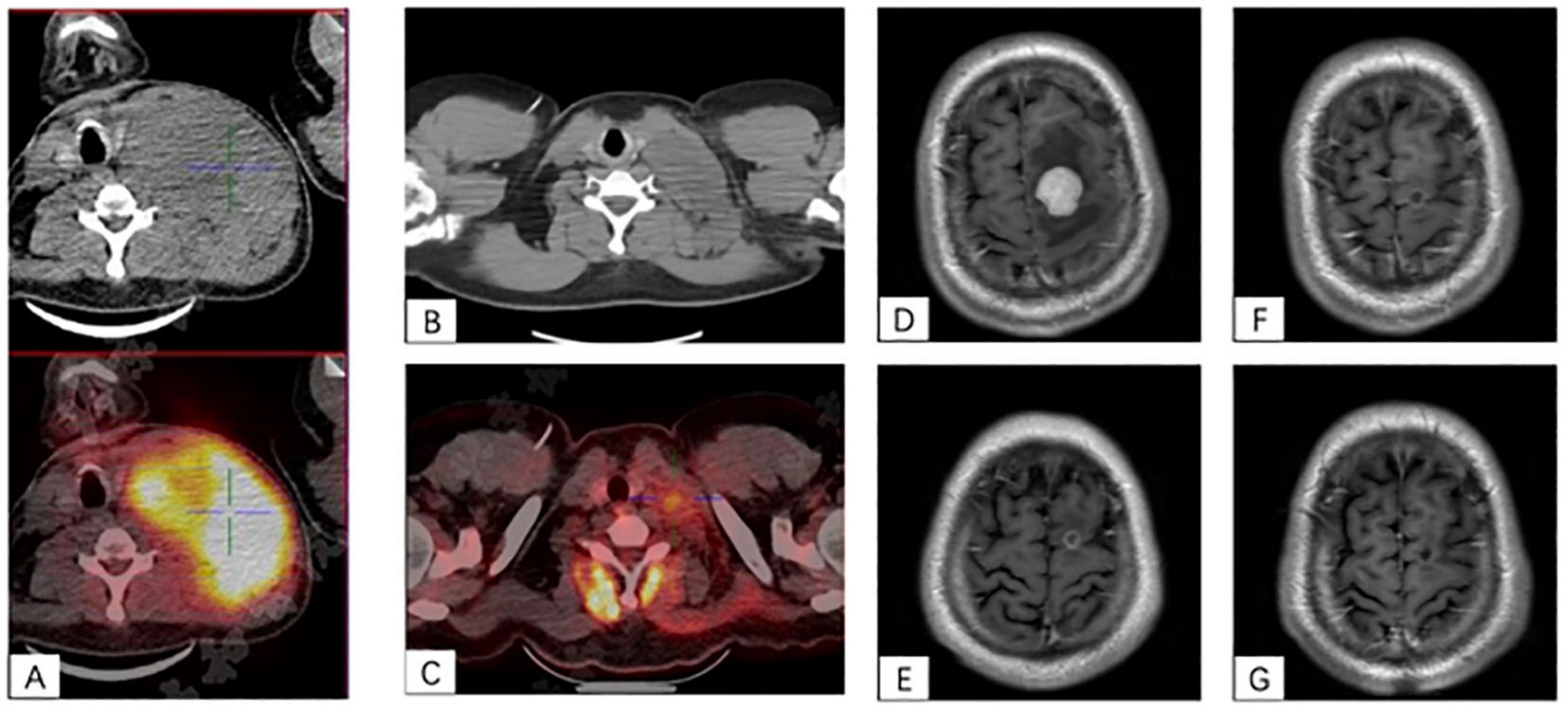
(A–C) A case of bulky left cervical disease. (A) Baseline PET/CT scan showed a 13.1 cm × 8.4 cm × 10.5 cm mass with SUVmax to be 20.5 at the left side of the neck and pushing the trachea to the right. (B) The mass quickly shrank to 6×2.6 cm after hyper-fractionated radiotherapy. (C) After CAR-T cell infusion at 1 month, PET/CT scan showed a 3.2 cm × 1.9 cm mass with SUVmax of 3.2. (D–F) A case of CNS lesion. (D) Baseline contrast-enhanced MRI showed a 2.5-cm enhanced nodule with peripheral edema in the left frontal lobe. (E) After radiotherapy, the lesion shrank to 0.75 cm with ring-like enhancement. (F) After CAR-T cell infusion at 1 month, the lesion was 0.5 cm with ring-like enhancement. (G) The lesion had no enhancement on the contrast-enhanced MRI at 3-month after CAR-T cell infusion.
